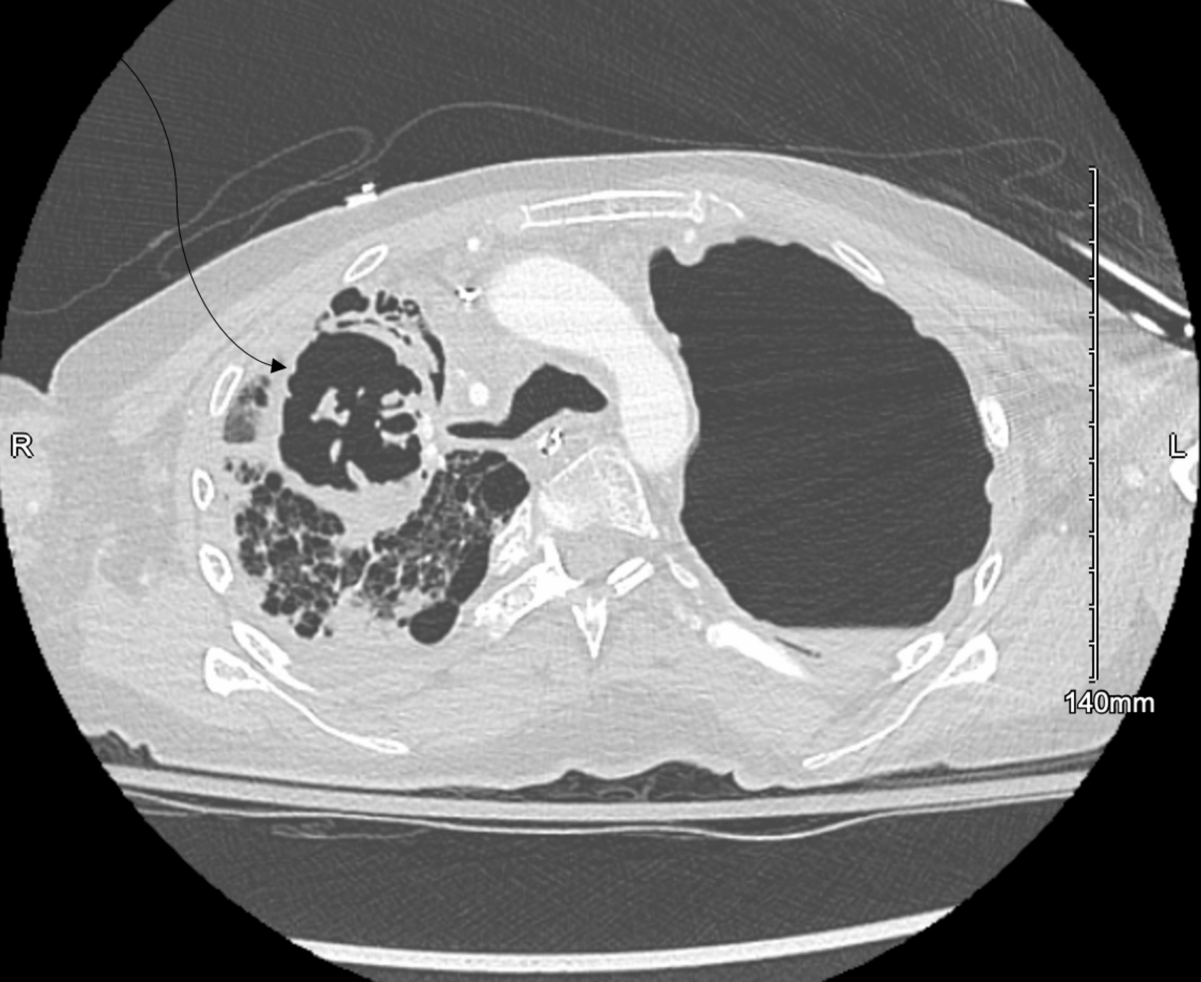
Follow-Up Computed Tomography Scan Demonstrating Further Progression of the Right Upper Lobe Cavitary Lesion The image shows a de-identified right upper lobe cavitary lesion in a patient with *STAT3*-deficient hyperimmunoglobulin E syndrome and secondary hypogammaglobulinemia. Compared with Figure 2, the cavitation shows increased complexity and wall thickening, suggestive of progression such as fungal infection or necrotizing pneumonia. No identifying patient information is present, and informed consent for publication was obtained.
